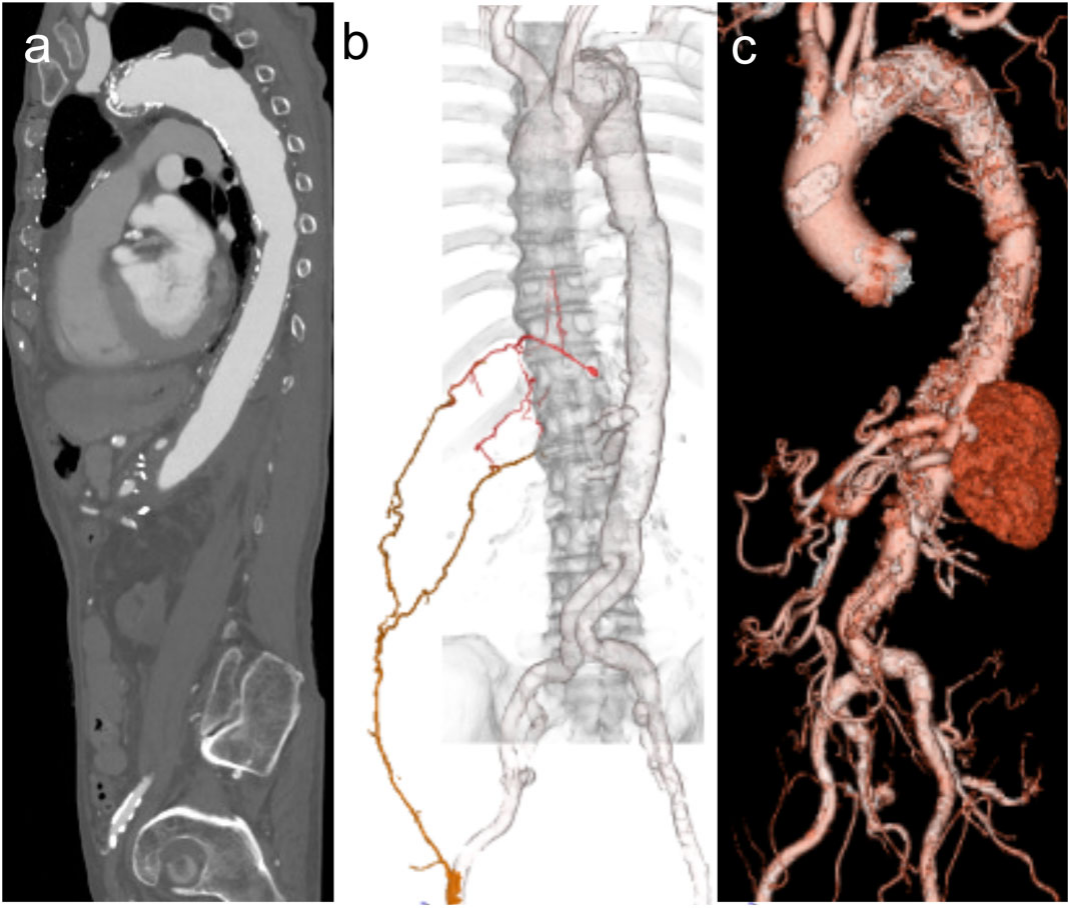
(a) Computed tomography angiography revealed an aneurysm in the distal aortic arch within the sagittal plane. (b) Collateral vessels to the Adamkiewicz’s artery via the right 11th intercostal artery from the right common femoral artery. (c) Postoperative three-dimensional computed tomography revealed no contrast effects into the aneurysmal sac and no access route injury.
